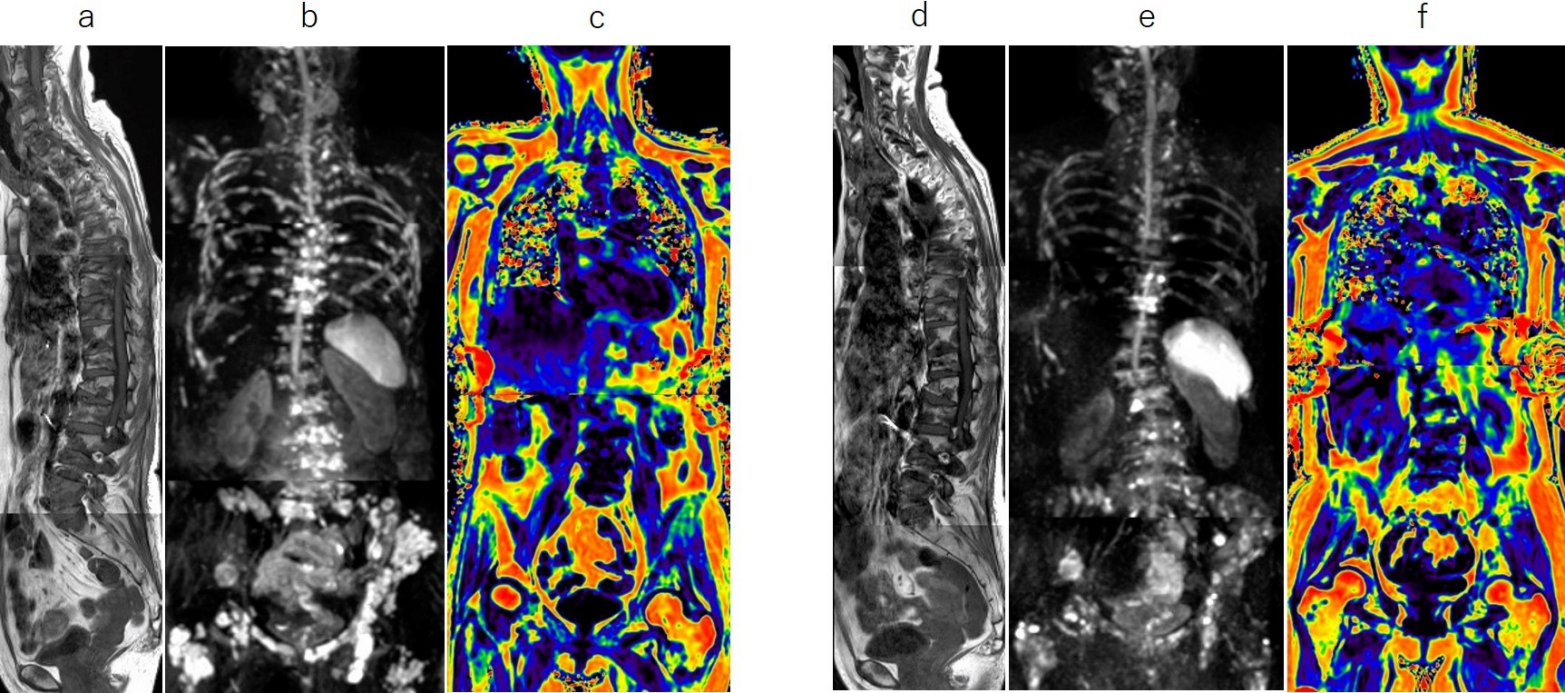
Whole-body MRI with a combined diffuse and focal pattern. Sagittal T1-weighted images (T1WI, a), whole-body diffusion-weighted MRI (WB-DWI, b), and fat fraction (FF) map (c) at baseline, and corresponding T1WI (d), WB-DWI (e), and FF map (f) at 2 cycles of chemotherapy for a 64-year-old woman with symptomatic myeloma who relapsed after chemotherapy with carfilzomib, lenalidomide, and dexamethasone. MRI shows focal bone lesions and heterogeneous low signal in the spinal bone marrow on T1WI (a). After 2 cycles of chemotherapy with pomalidomide and dexamethasone, total diffusion volume has decreased (from 263 ml to 176 ml), but FF in lumbar bone marrow has not shown significant change (from 15% to 16%).
